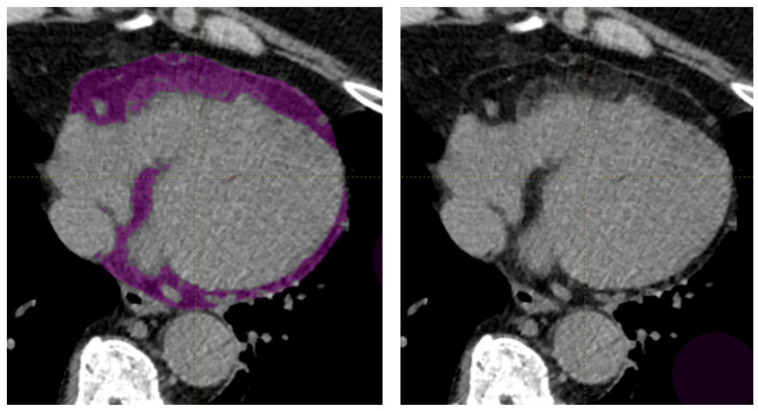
Epicardial adipose tissue (EAT) segmentation on cardiac CT. The EAT volume is highlighted in purple (left panel). The unsegmented image is shown on the right panel.
